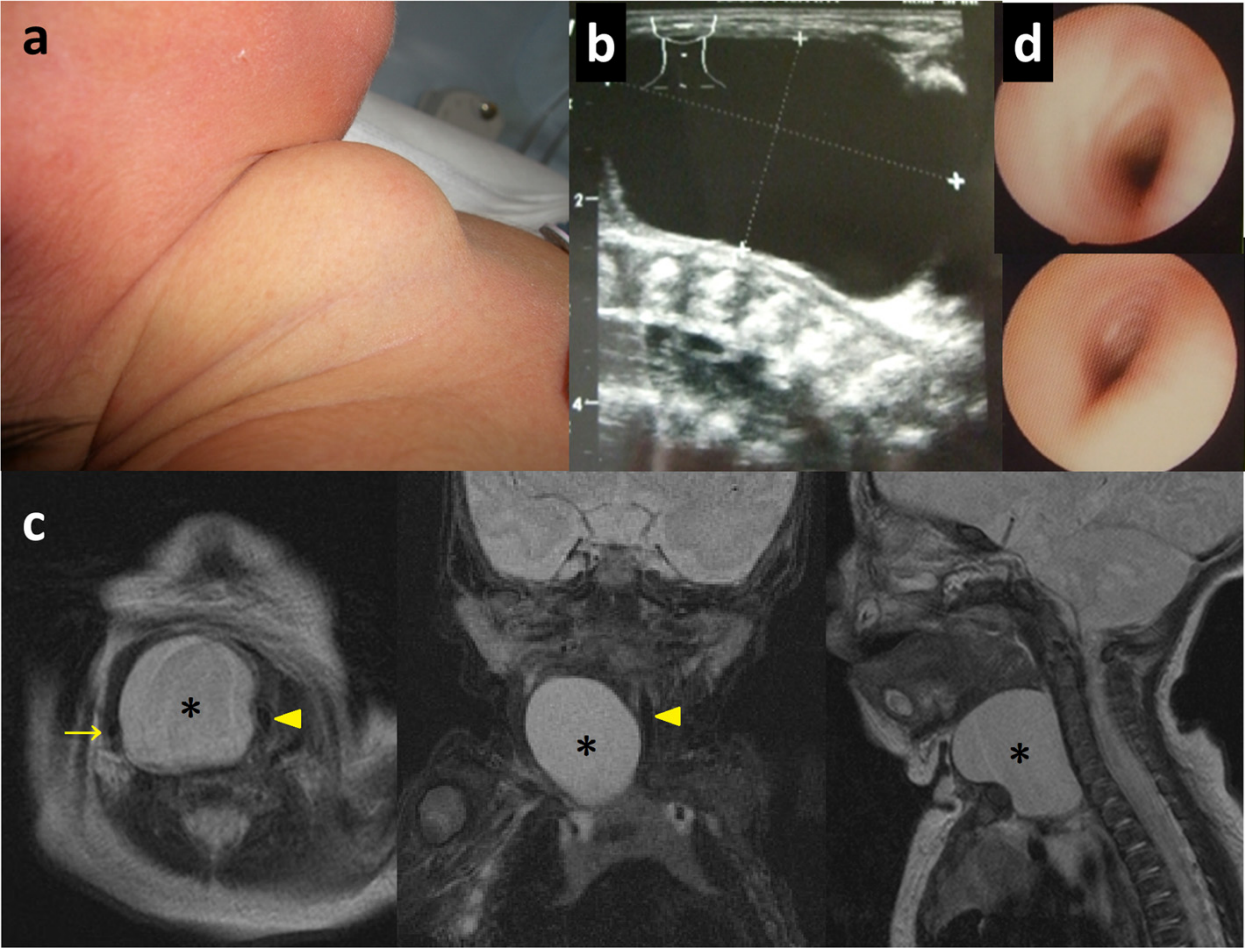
Clinical findings in a newborn infant girl with esophageal duplication cyst. a On physical examination, there was anterior neck swelling on the right. b Ultrasonography showed a simple cyst measuring 40 × 24 × 33 mm in the right neck. c T2-weighted enhanced magnetic resonance imaging revealed a simple cyst (*asterisk*) that displaced the sternocleidomastoid muscle anteriorly, the carotid sheath (*arrow*) to the right, and the larynx and trachea (*arrow head*) to the left. d Bronchoscopy showed compressive deformation of the trachea from the subglottis to the carina
